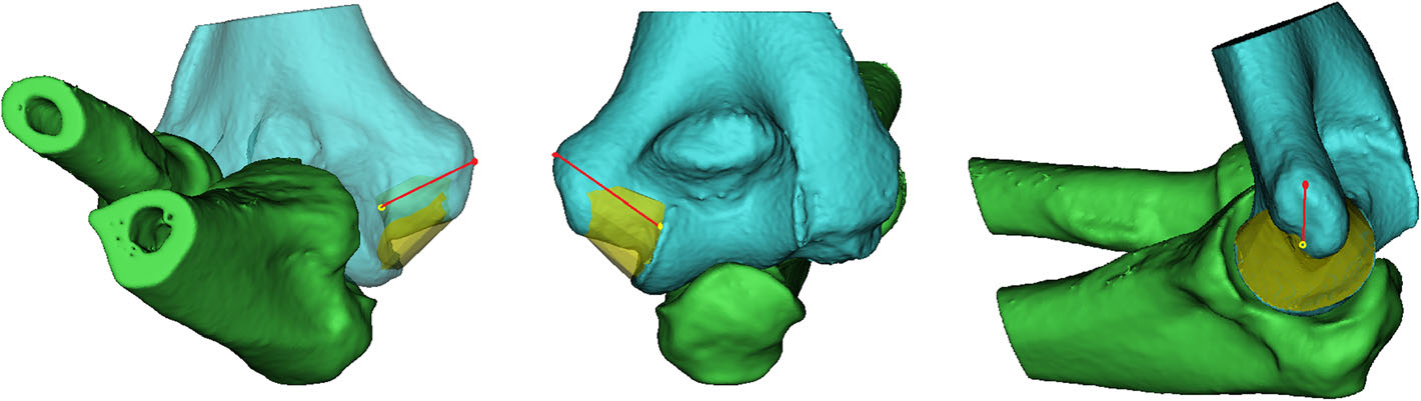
The elbow, reconstructed by Materialize Mimics software using CT images. Yellow area represents the cubital tunnel; red dot, apex of the medial epicondyle (AME); yellow dot, the center of trochlear border (CTB). The red line connecting the two points represents the vertical axis of bony cubital tunnel (ABT)
